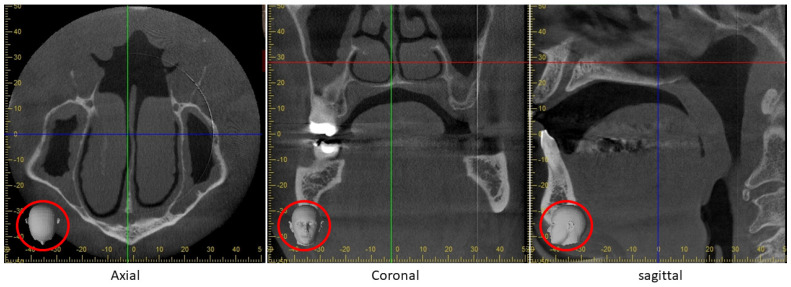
A large FOV CBCT, which includes not only the whole jaws, but also the clivus, most of the antro-nasal complex, the vertebral column and the nasopharynx. The standard orthogonal planes are displayed. They are also represented by the red-ringed heads. The positions of these planes are axial (red line), coronal (blue line) and sagittal (green line). Note also the metal of the dental restorations attenuates the primary beam at the level of the occlusal plane (coronal and sagittal planes). This artifact extends through the whole thickness of the head at that level, including the odontoid process (dens) of the second cervical vertebra (just below the anterior arch of the first cervical vertebra).
